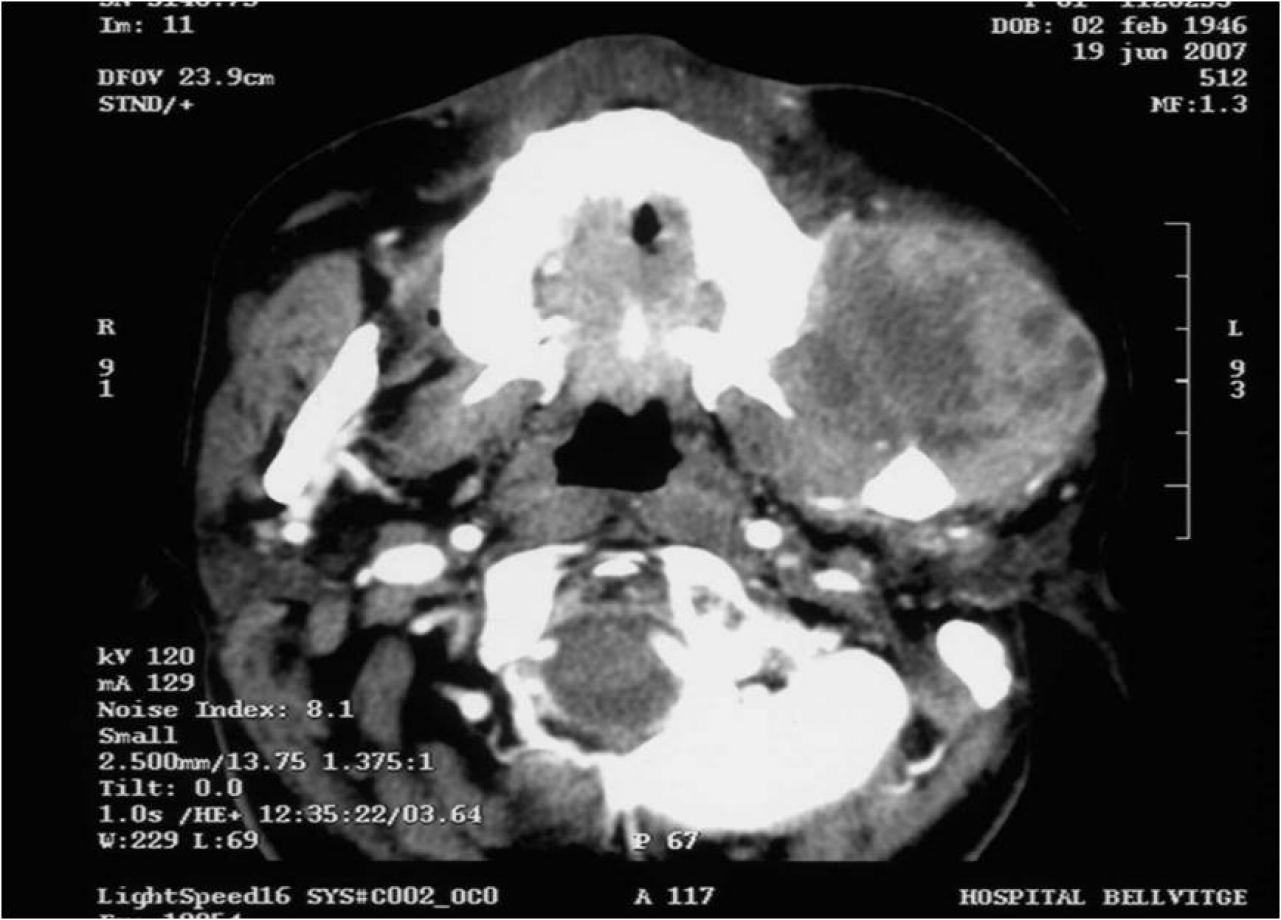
Pre-treatment axial cervical contrast-enhanced computed tomography scan. The scan shows a bulky, expansive, soft-tissue mass at the masticator space with heterogeneous enhancing and central necrosis. The infiltration of the masseter and medial pterigoid muscles is seen.
